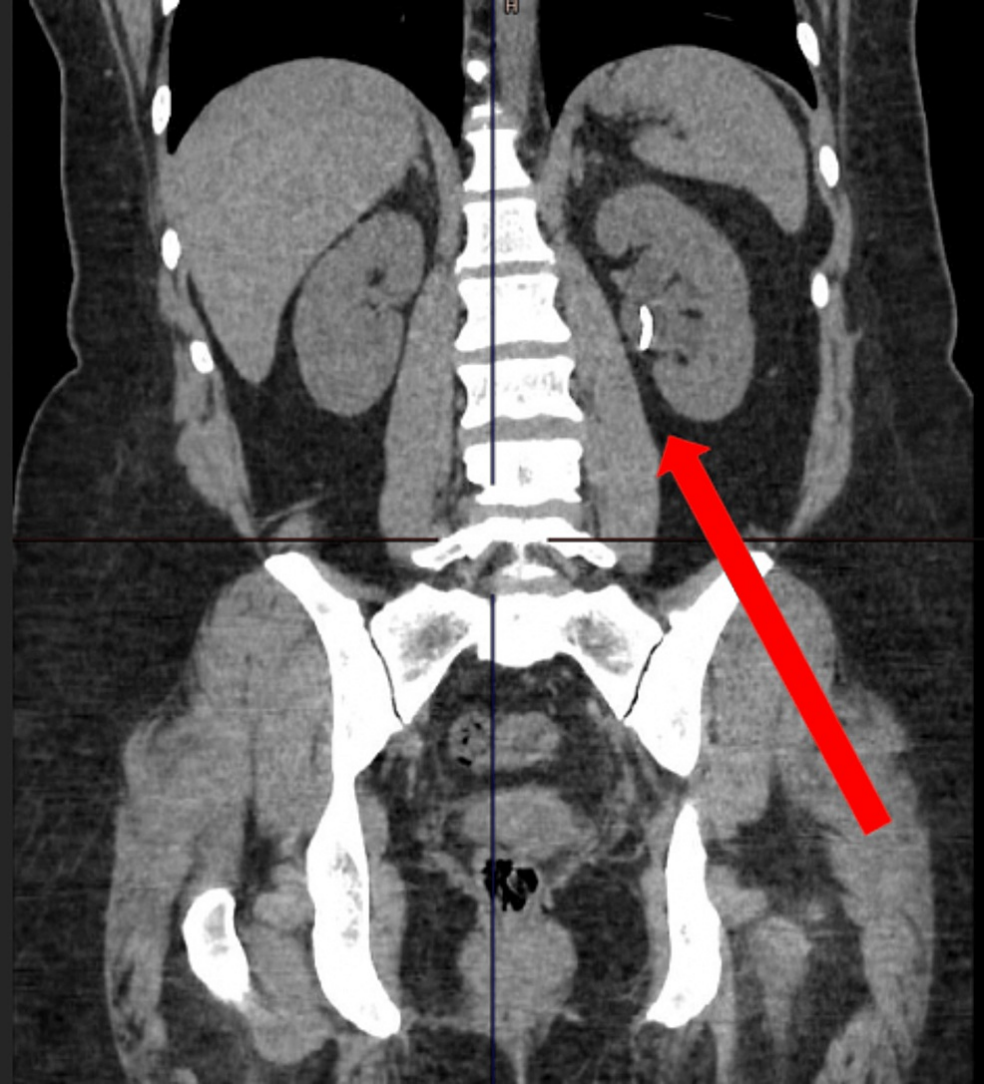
Computed tomography urogram showing resolution of the left hydroureteronephrosis
